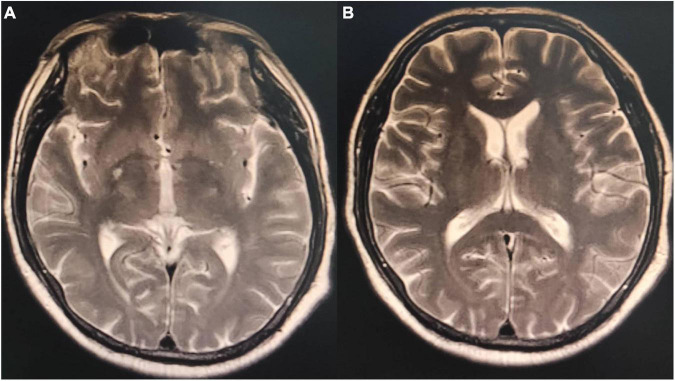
Magnetic resonance imaging T2 phase of the patient’s brain. (A) Small patches of the long T2 signal shadow in the right basal ganglia with hyperintensity in T2 fluid-attenuated inversion recovery (FLAIR) and a few patchy long T2 signals in the white matter of bilateral cerebral hemispheres with hyperintensity in T2 FLAIR. Acute lacunar infarction in the right basal ganglia might be large, and there were few ischemic degeneration foci in the white matter of the bilateral cerebral hemispheres. (B) Small patches of long T2 signal shadow were observed in the right basal ganglia, with slight hyperintensity in T2 FLAIR. A few patchy long T2 signals were observed in the white matter of the bilateral cerebral hemispheres, with hyperintensity in T2 FLAIR. A few ischemic degeneration foci in the white matter of bilateral cerebral hemispheres and ischemic infarction foci in the right basal ganglia were considered.
